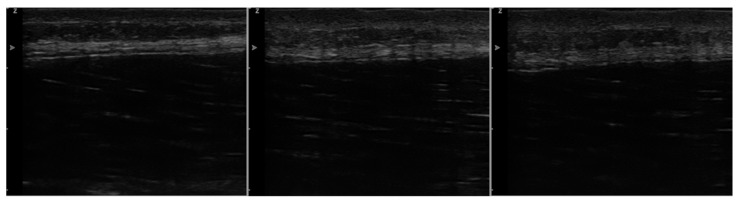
Observed changes in the area of the superficial fascia before instrument-assisted manual therapy (IAMT) intervention (left), immediately after IAMT intervention (middle), and 45 min after IAMT intervention (right) on the ultrasound images.
